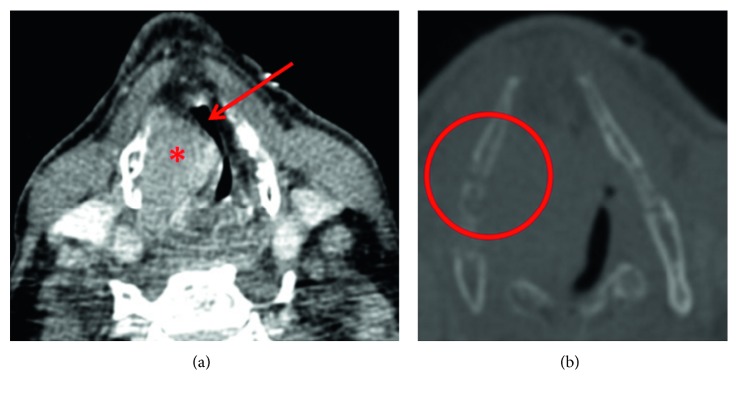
CT scan of the patient with the extramedullary multiple myeloma of the larynx. The tumor displays as a solid mass in the area of the right vocal cord (red asterisk). The lumen of the larynx and therefore also the airway is extremely narrowed (red arrow) (a). The thyroid cartilage on the right side seems to be significantly thinned out (red circle) (b).
